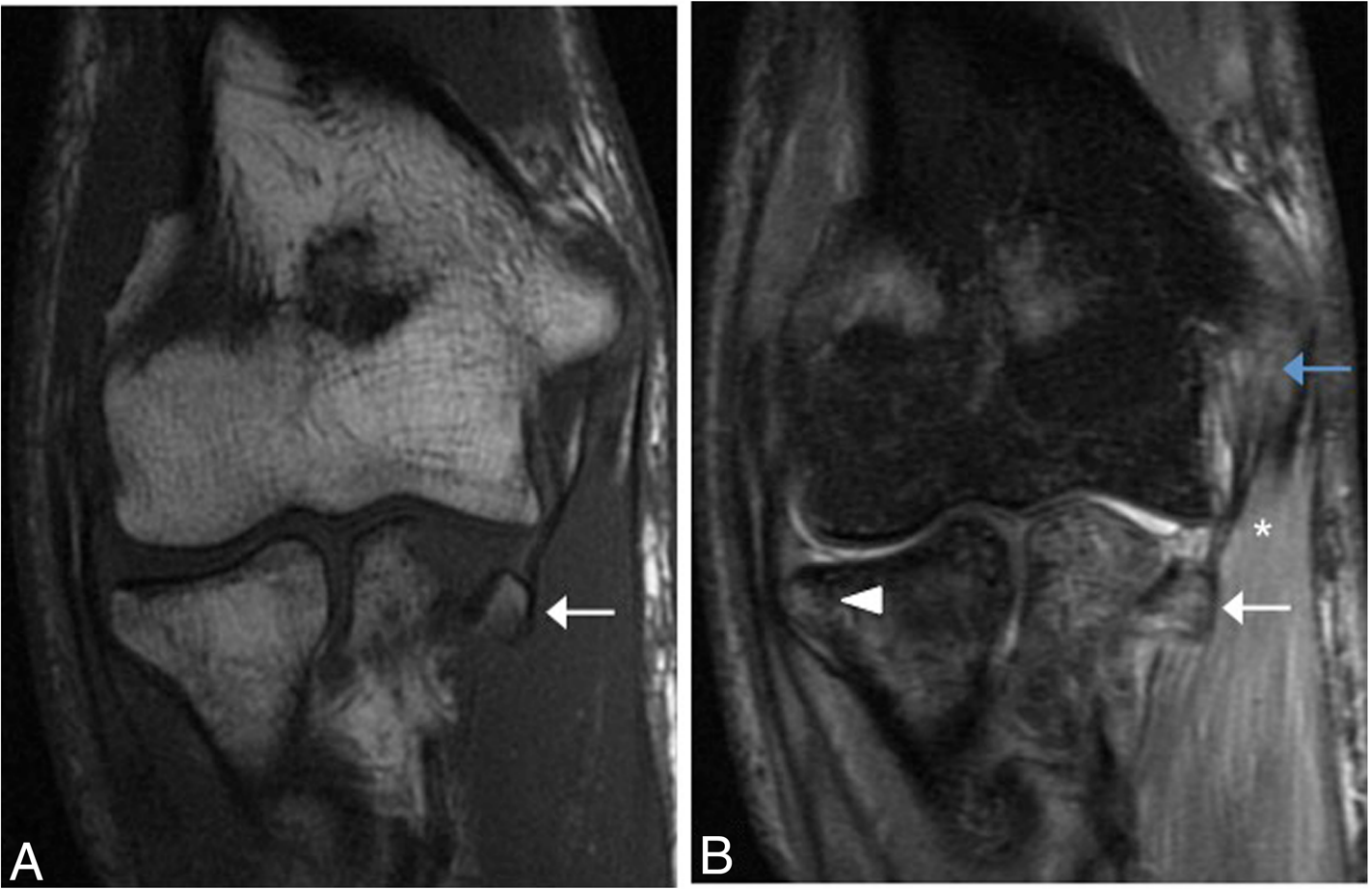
A 32-yer-old man with pain and medial instability after a fall on the outstretched arm. Coronal T1-weighted MRI (a) and coronal FS PD-weighted MRI (b) showing an acute avulsion fracture of the anterior bundle of the medial collateral ligament insertion in the sublime tubercle (white arrows), acute partial-thickness proximal tear of anterior bundle of the medial collateral ligament (blue arrow), flexor digitorum superficialis muscle edema (white asterisk), and radial head contusion (white arrowhead)
